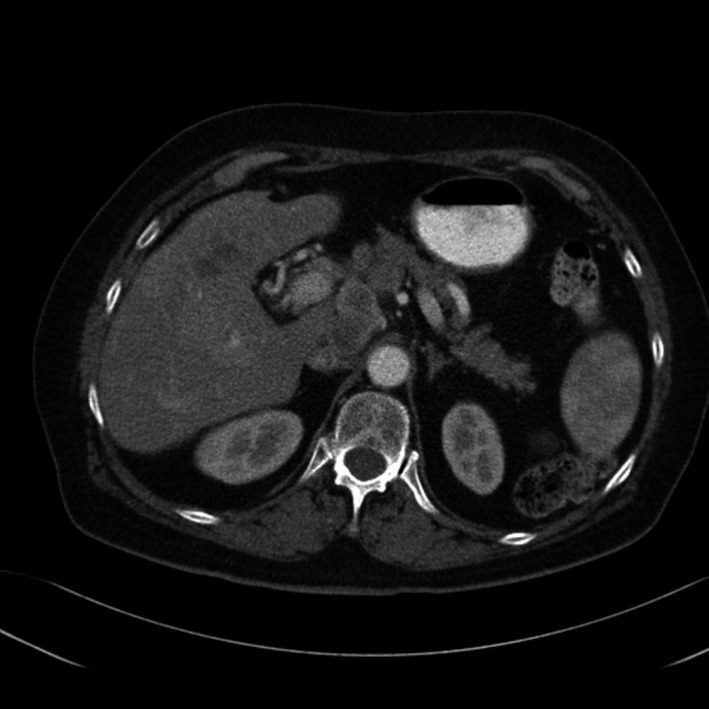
Patient 2—Baseline CT image arterial phase. This patient's the tumor at baseline (prior to trial enrollment) was measured at 3.1 X 4.2 cm in segments 4b and 5.
